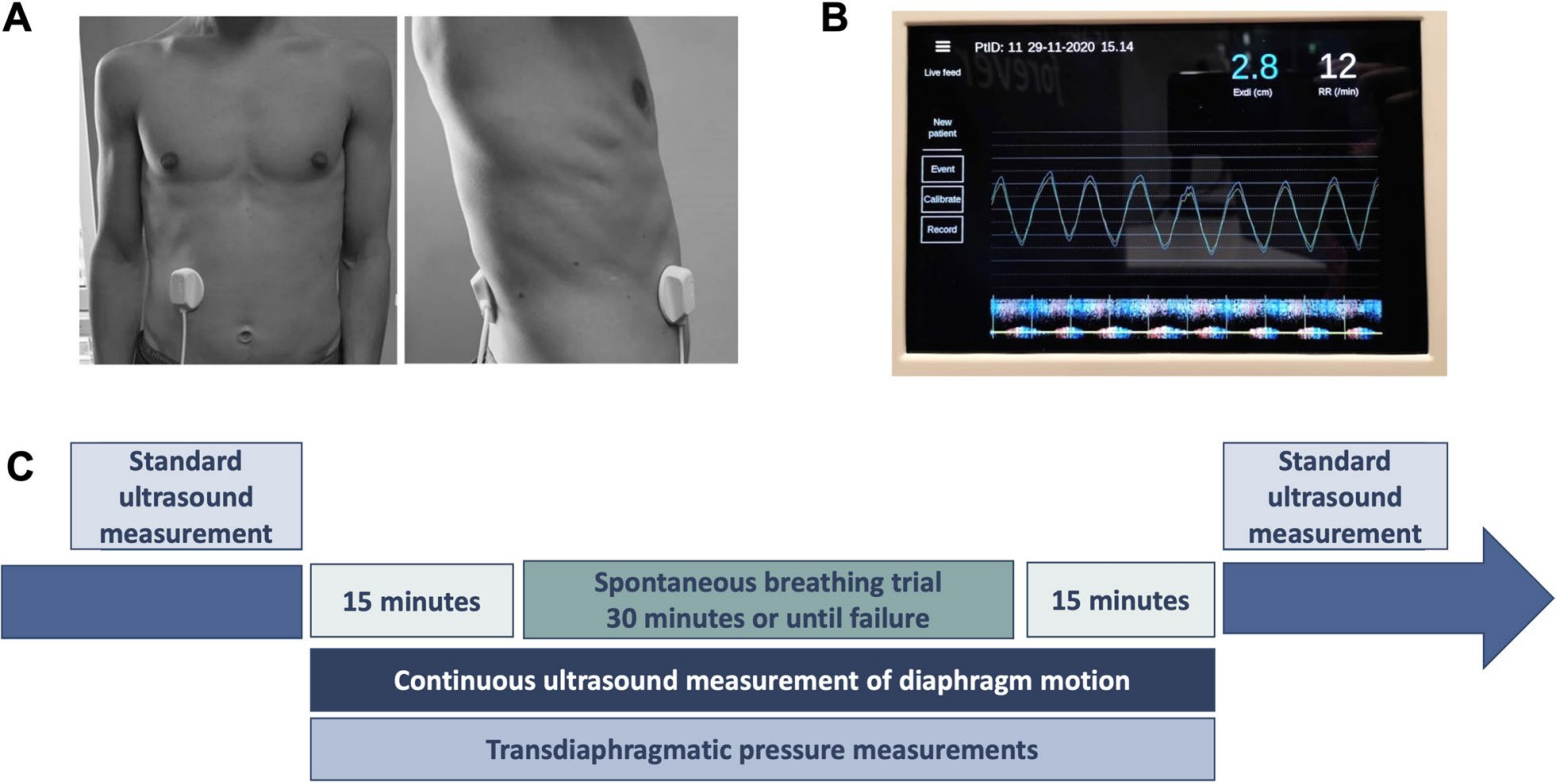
Continuous ultrasound diaphragm excursion and velocity monitoring probe placement and screen. Panel A. *The anterior sensor was placed along the right mid-clavicular line with the upper border of the sensor approximately 2 cm below the lowest rib. The posterior sensor was placed on the back of the patient directly opposite to the anterior sensor*. Panel B. *The screen displays the continuous diaphragm excursion curve as well as the calculated diaphragm excursion in cm (EXdi) and respiratory rate (RR) updated for each breath. The yellow line represents the raw ultrasound signal, and the blue line represents the ultrasound signal compensated for the natural up and down movement of the abdomen during breathing. At the bottom of the screen, a live M-mode plot of diaphragm velocity is displayed. Panel C.* First, a standard ultrasound recording of diaphragm maximal excursion and veloocity (EXdi and PCVdi, 10 cycles each) was performed. Of notice, because of interferences between the ultrasound waves produced by the continuous ultrasound measurement (CUSdi) and the standard ultrasound probes and because the two probes cannot be positioned together at the same location on the abdomen, the two measurements could not be done simultaneously. As such, immediately after the standard ultrasound recording, the sensors for CUSdi were attached and CUSdi recording of diaphragm excursion and velocity was performed. In parallel, the double-balloon catheter was positioned and transdiaphragmatic pressure was continuously measured with. After a 15-min recording of CUSdi and Pdi, the spontaneous breathing trial was initiated, for 30 min or until failure. The recording of CUSdi and Pdi was continued 15 min after the end of the SBT. Right after that, the sensors for CUSdi were removed and a second set of standard ultrasound recording of EXdi and PCVdi (10 cycles each) was immediately performed
